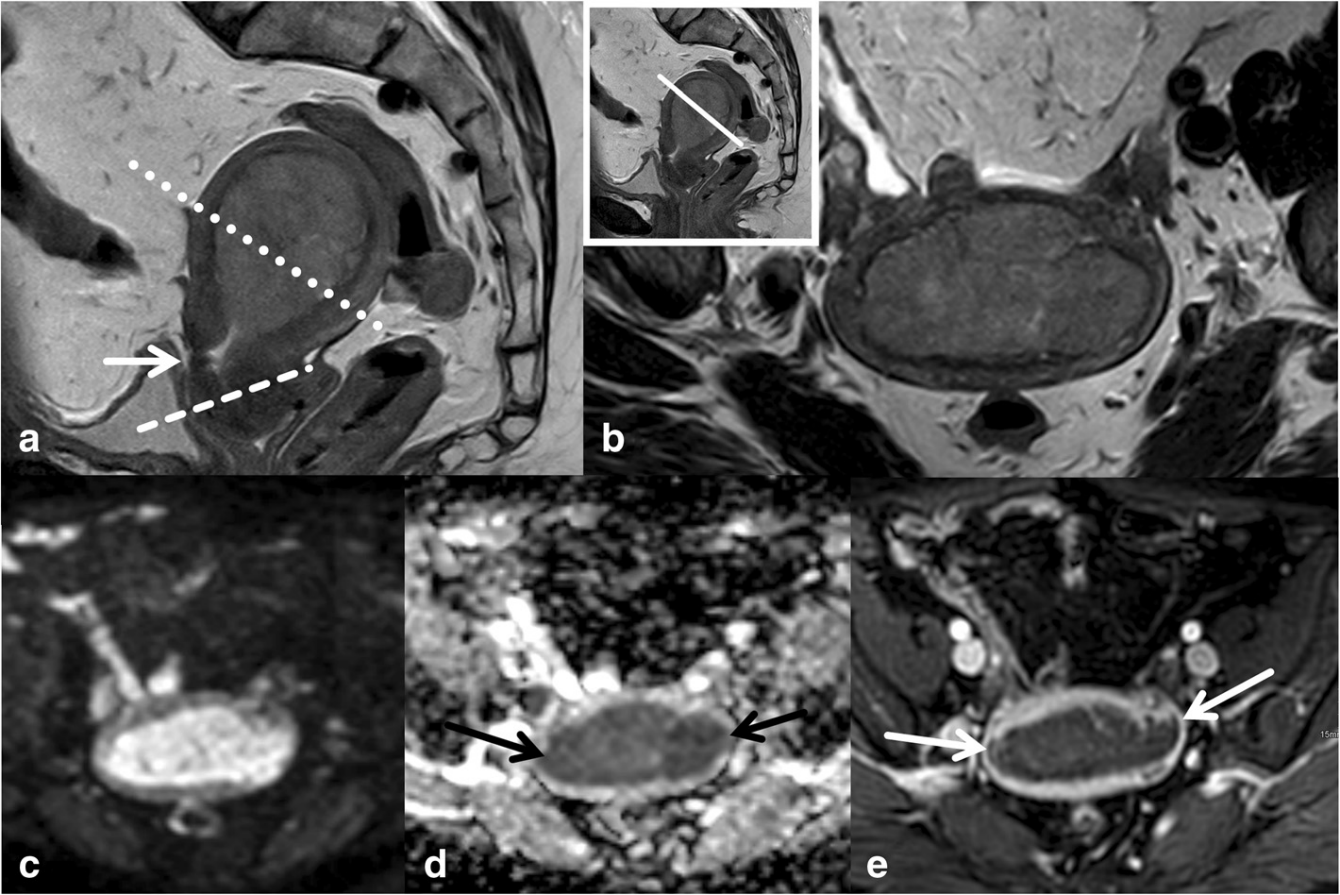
Value of complementary DWI and GdT1 to assess myometrial invasion. Endometrial cancer expanding the uterine cavity and extending to the upper cervix (arrow) is seen on sagittal T2WI (a). The planes for assessing myometrial and cervical invasion are perpendicular to the long axis (dotted and dashed lines, a). T2WI perpendicular to the uterine cavity (b) demonstrates thinning of the myometrium. Deep myometrial invasion can be confirmed by DWI (b = 1000) (c) and (d) and by Gd T1 FS (e)
